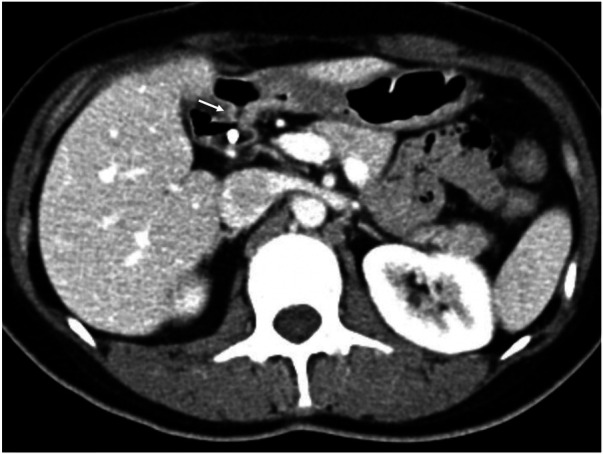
Axial view of post-ERCP enhanced abdominal CT detected the choledochoduodenal fistula in the proximal descending duodenum (white arrow), with implanted biliary stent. ERCP: endoscopic retrograde cholangiopancreatography.
